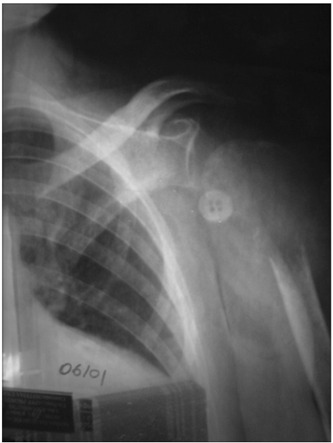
Radiograph of the left shoulder showing a pathological fracture of the proximal humerus. Note an osteolytic lesion located in the meta epiphyseal region of the bone.
